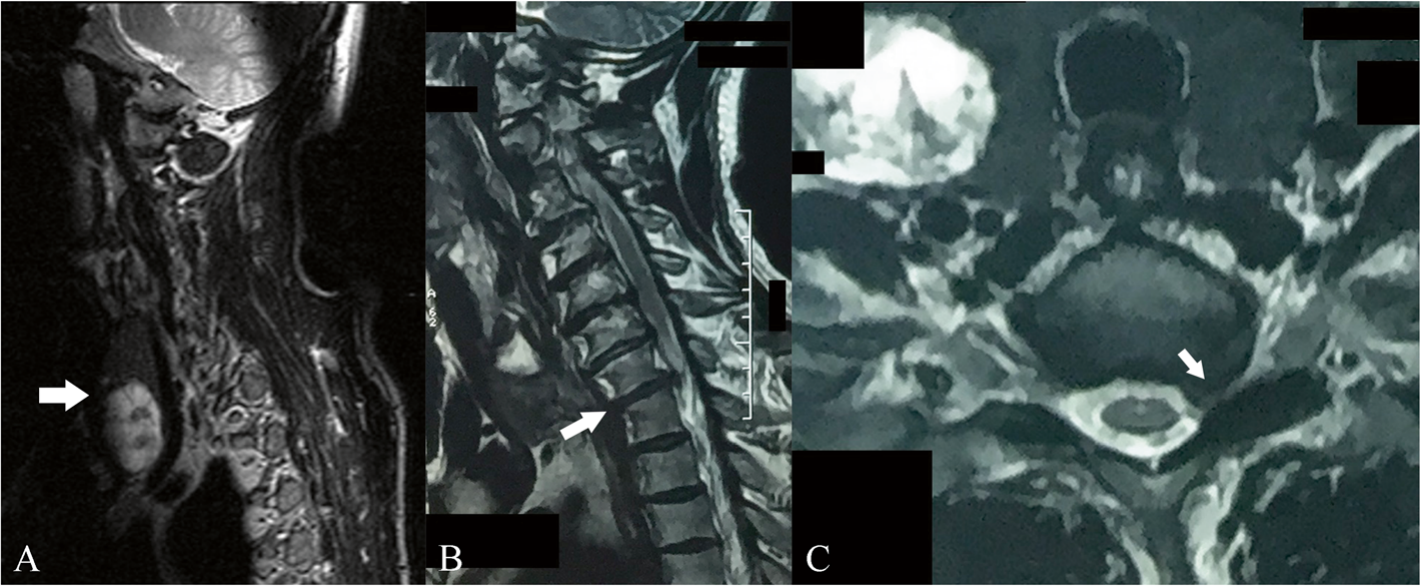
Pre-operative MR images. A T2 weighted sagittal image showing the enlarged right thyroid gland (white arrow) (a). A sagittal T2 weighted image at C7-T1 level (white arrow) (b) and an axial T2 weighted image demonstrating a compressed left root at the same level (white arrow) (c)
